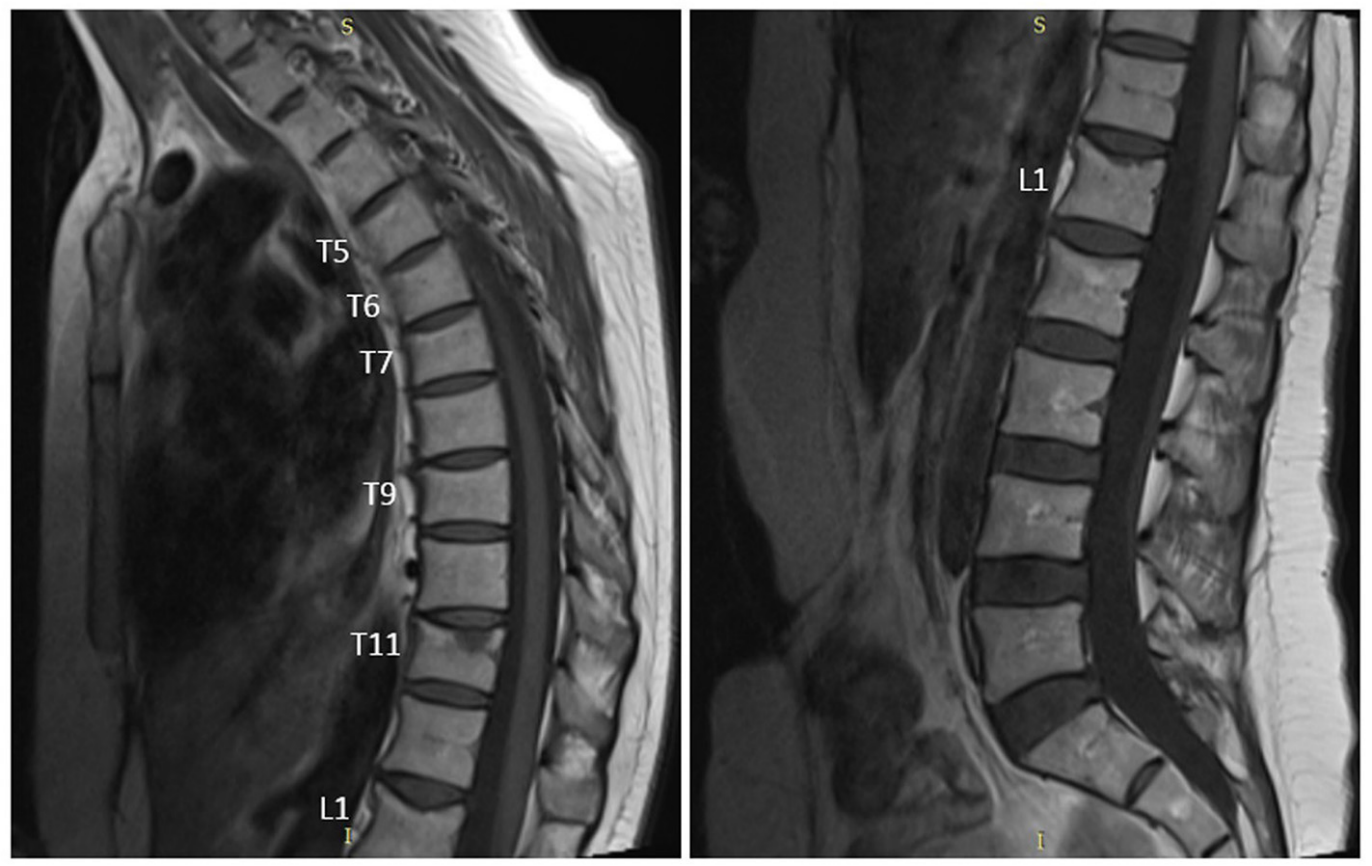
Sagittal T1 magnetic resonance images of the thoracic and lumbar spine shows acute bone marrow edema at T11 vertebra and loss of height at T5–7, 9 and L1 vertebrae (height loss ratios at T5, T6, T7, T9 and L1 are 24%, 25%, 23%, 24%, 27% respectively).
